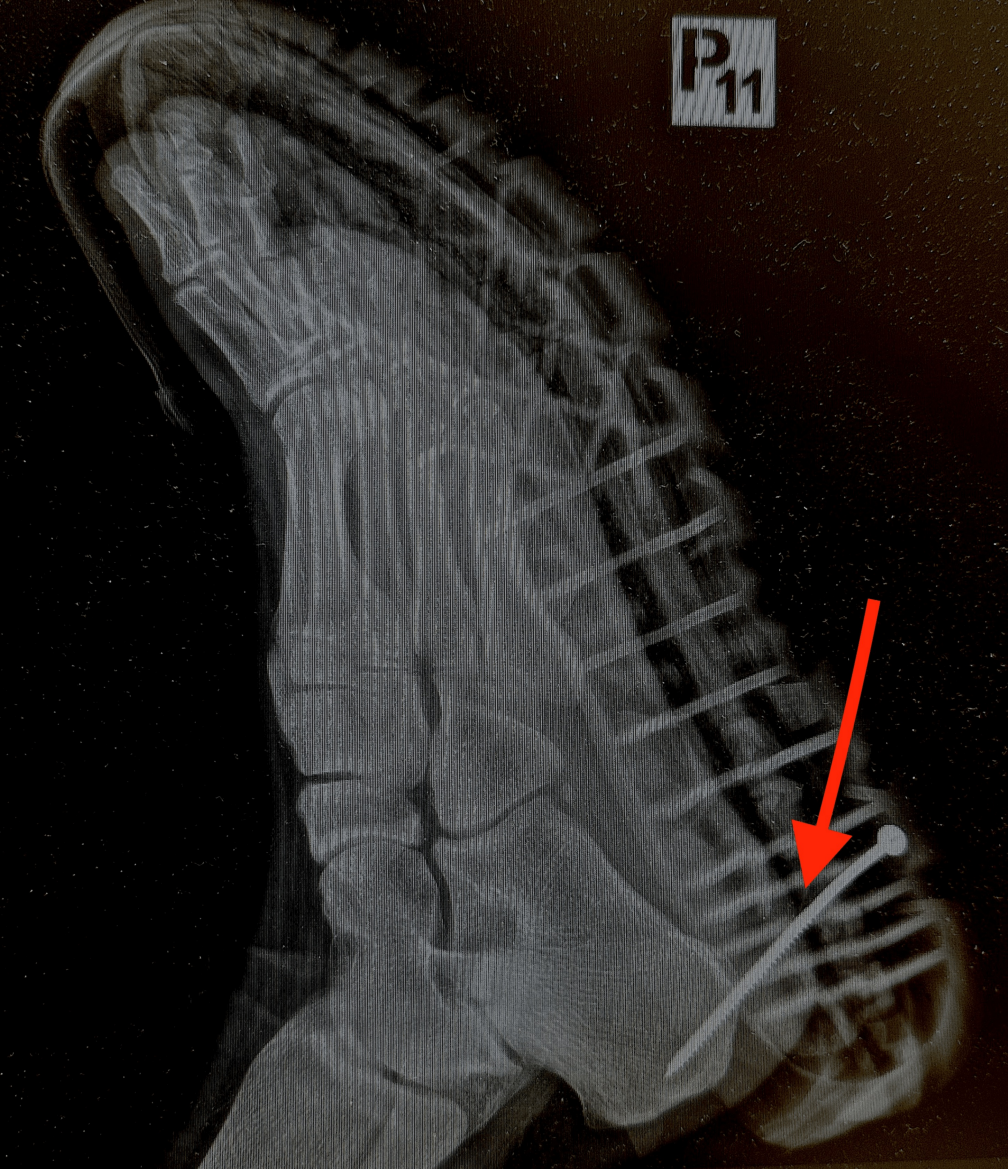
Pre-removal radiograph demonstrating wood screw penetration. Lateral radiograph of the foot showing a metallic wood screw penetrating the plantar soft tissues without evidence of osseous involvement. The red arrow indicates the position of the screw.
